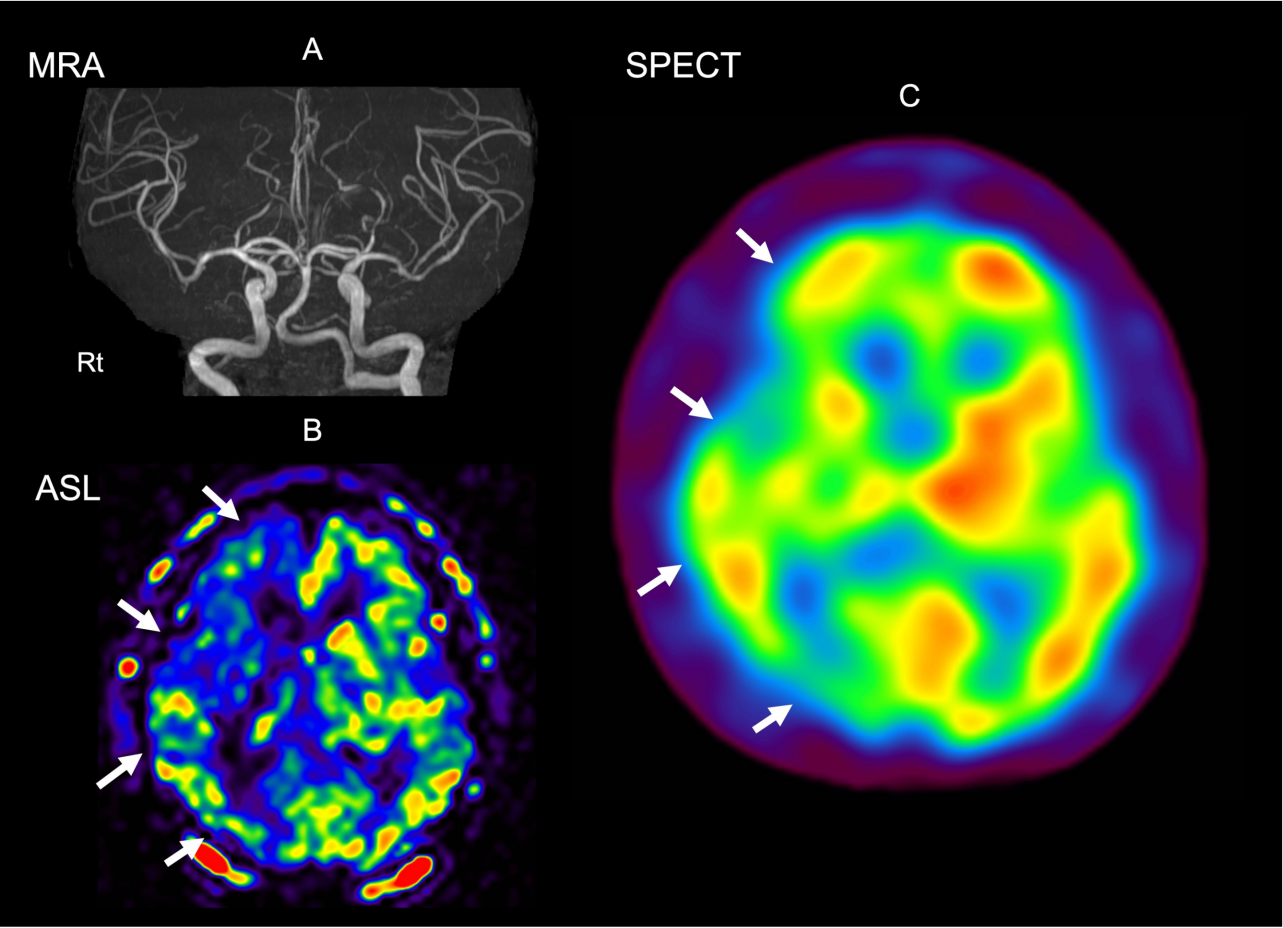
ASL perfusion MRI and IMP-SPECT ASL: arterial spin labeling; MRA: magnetic resonance angiography; IMP-SPECT: N-isopropyl-p-[123I]iodoamphetamine single-photon emission computed tomography (A-B) Day 6 head MRI images. (A) The left image is an MRA image. (B) ASL image. While there is no difference in blood flow, extensive reduced blood flow is observed in the right cerebral hemisphere. The white arrow indicates the area with reduced blood flow. (C) Brain blood flow scintigraphy SPECT (¹²³I-IMP). Reduced blood flow is observed in the transition zone between the right frontal lobe and parietal lobe, and in the right occipital lobe. Red indicates increased blood flow; blue indicates decreased blood flow. The white arrow indicates the area with reduced blood flow
